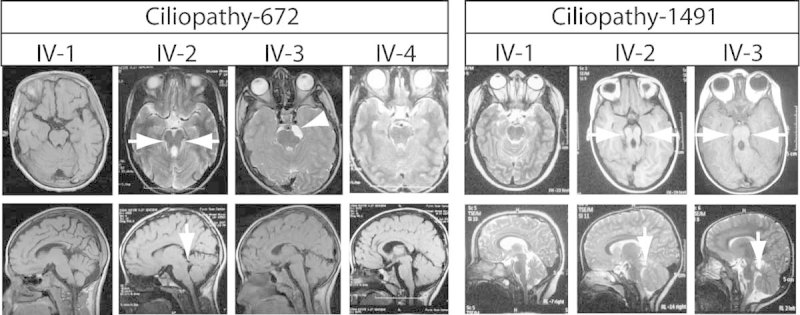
Brain MRI of affected members of both families (axial view at the midbrain-hindbrain junction (top) and midline sagittal (bottom)). In Ciliopathy-672, MTS is evident only in IV-2 (arrows), and is absent in IV-1, -3, and -4. There is a small arachnoid cyst evident in IV-3 (arrowhead), not seen in the other members. Consistent with the axial views, only IV-2 shows a horizontally oriented superior cerebellar peduncle (arrow). Despite the absence of the MTS, there is mild cerebellar affection at lest for IV-1. In Ciliopathy-1491, MTS is evident in IV-2 and -3 (arrows) evidence by the horizontally oriented superior cerebellar peduncle (arrows). In IV-1 there is mild cerebellar vermis hypoplasia without the MTS.
